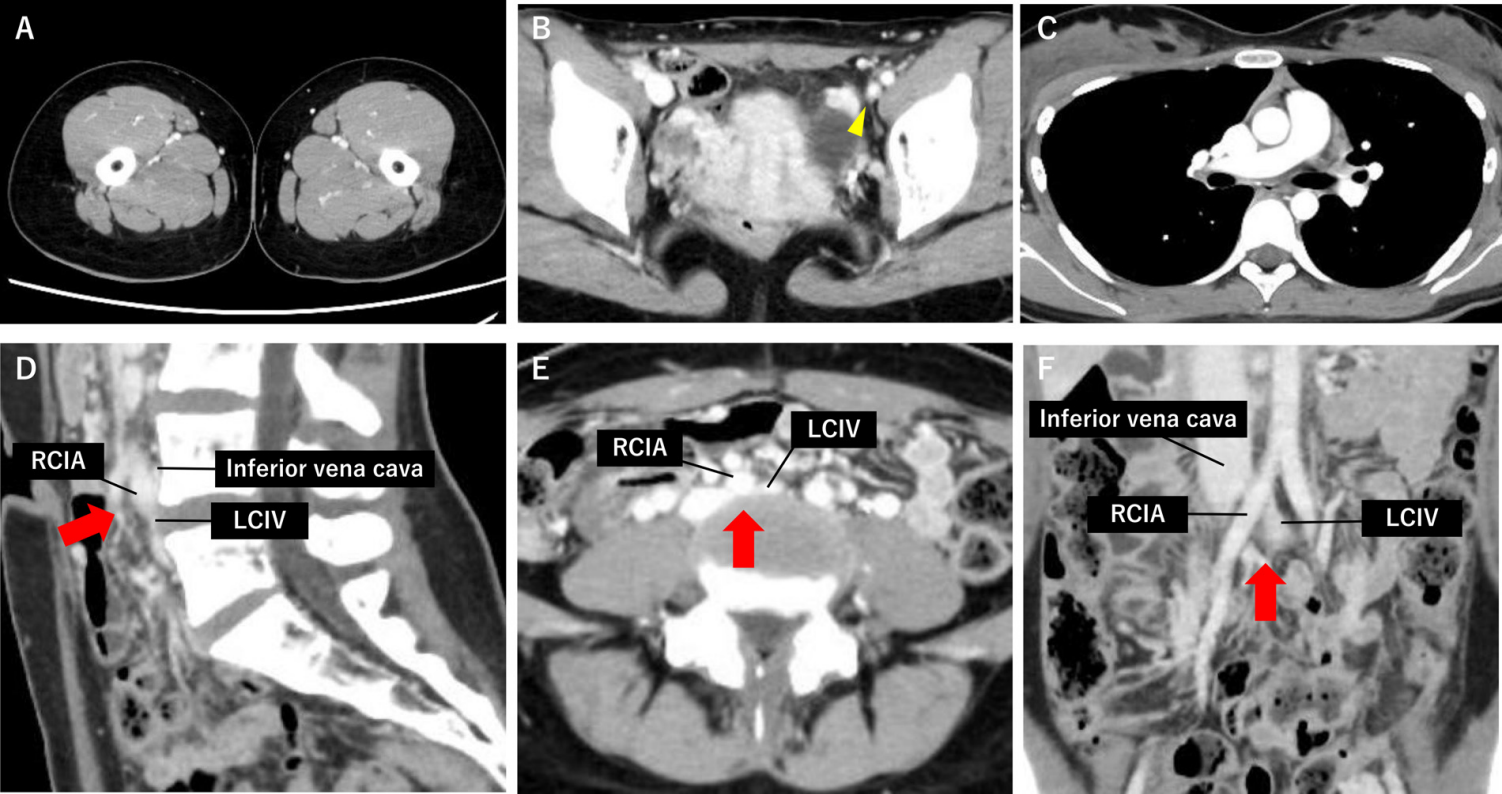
CT angiography performed after 2 months of anticoagulation therapy. (A) Improved left leg edema. C, Right pulmonary arterial thrombus is resolved. (B and D-F) There is a near-complete resolution of thrombi from the left common iliac vein (LCIV) to the common femoral vein (yellow arrowhead). Persistent LCIV compression between the right iliac common artery (RCIA) and the fourth lumbar vertebra is still evident (red arrows).
